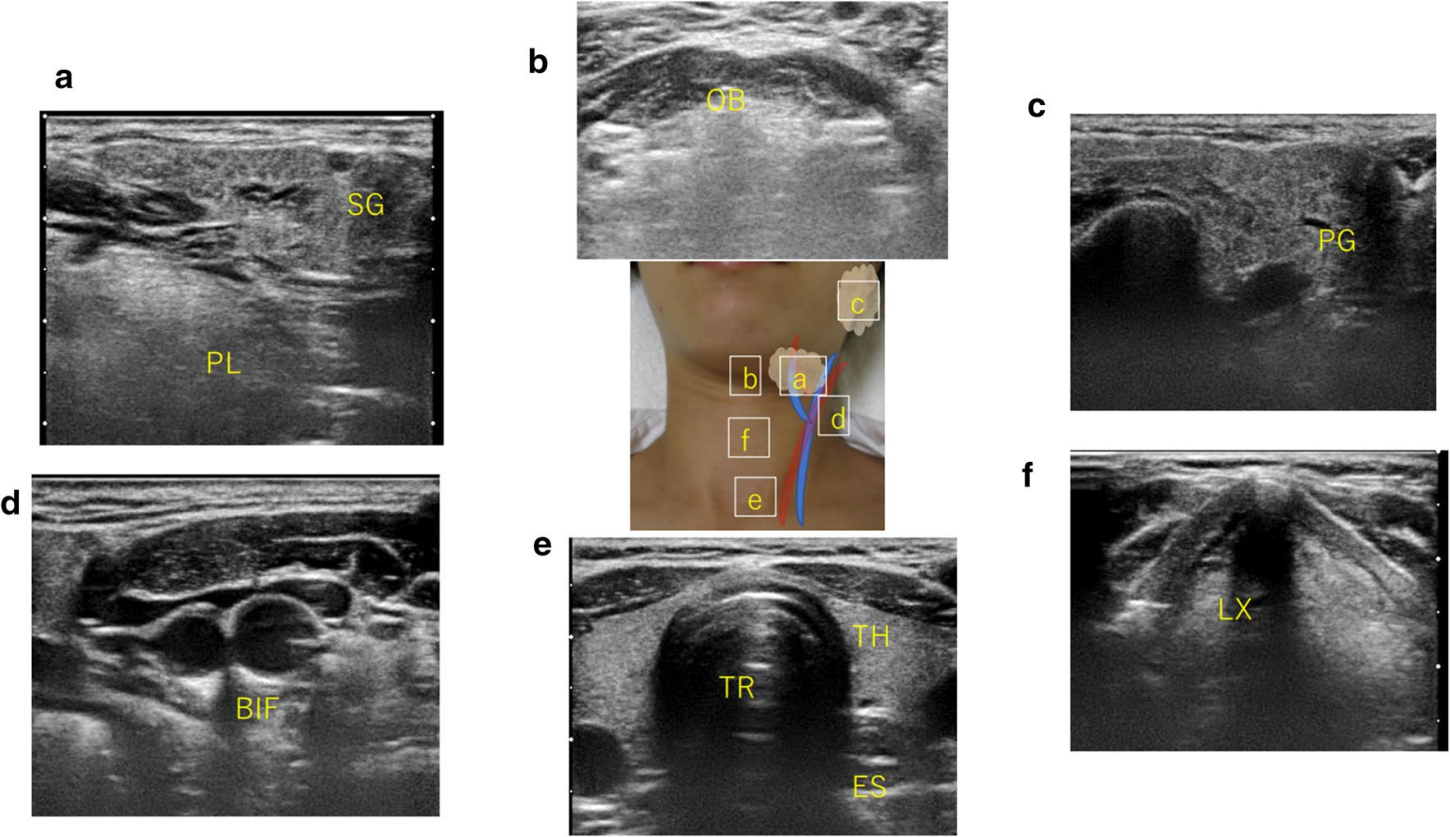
Basic image of the neck (systematic cervical ultrasonography). *SG* submandibular gland, *PL*: pharyngeal lateral wall, *OB*: oral base, *PG*: parotid gland, *BIF*: carotid bifurcation, *TR*: trachea, *TH*: thyroid gland, *ES*: esophagus, *LX*: larynx. The probe should be continuously scanned to ensure that it passes through these sites, and the left and right necks should always be observed. Observe in a manner that does not overlook changes in lymph nodes, blood vessels, and muscles along the way. a Submandibular region (submandibular gland, facial arteriovenous, oropharyngeal sidewall). b Submental region (oral floor muscles, base of tongue). c Parotid region (parotid gland, mandible, masseter muscle). d Carotid bifurcation (external carotid artery, internal carotid artery, internal jugular vein). e Anterior neck (thyroid, common carotid artery, internal jugular vein, cervical esophagus). f Larynx
